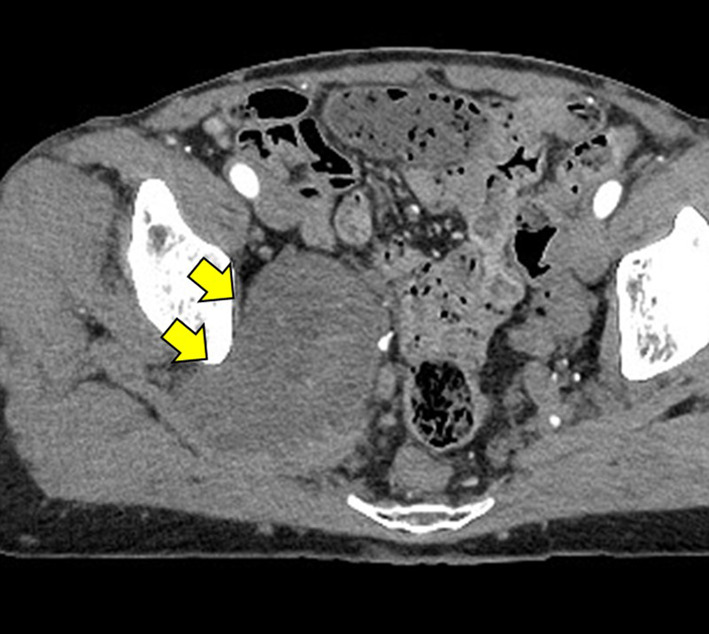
Pelvic CT. Pelvic CT shows a heterogeneous, internal, 75-mm-diameter mass in the right greater sciatic foramen
